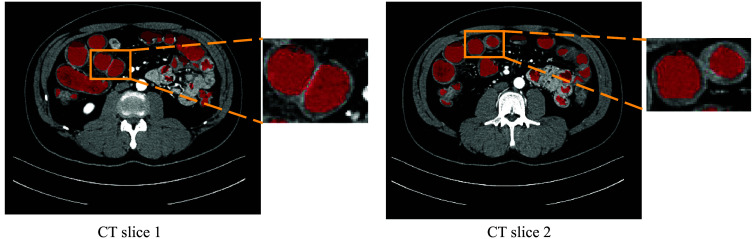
Example of CT slices. We enlarge some regions in the yellow boxes and see some contact in the intestine wall although just segmenting lumen regions can avoid this contact. In the enlarged parts, the red and light regions denote the lumen and wall, respectively.
